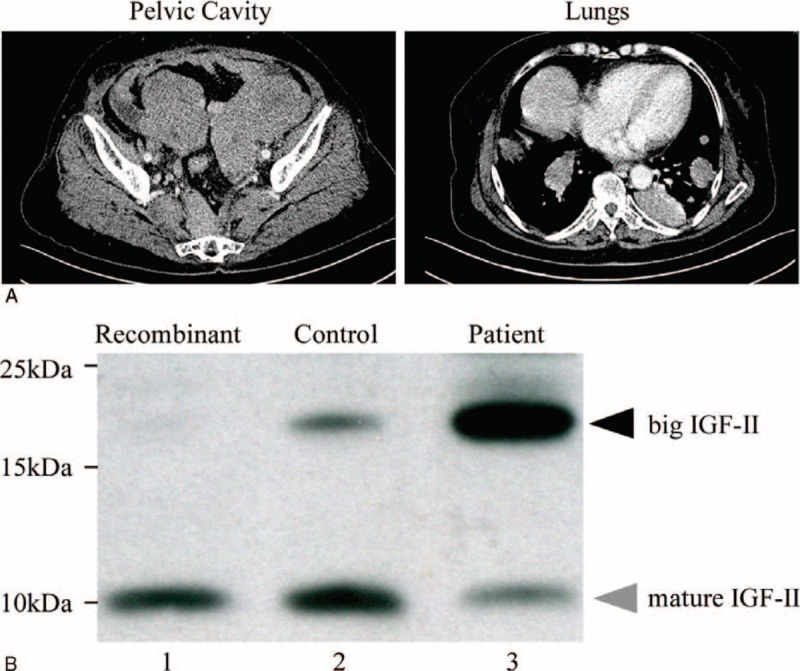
Diagnosis of NICTH. A: Abdominal CT scan revealed the pelvic recurrence and pulmonary metastases. B: Lane 3 showed that IGF-II was mainly detected as high molecular weight band in the sample from the patient, and lanes 2 and 3 showed the concentration of total IGF-II (ie, mature and big) of the patient's serum was higher than that in the control subject. CT = computed tomography; IGF-II = insulin-like growth factor II; NICTH = nonislet cell tumor hypoglycemia.
